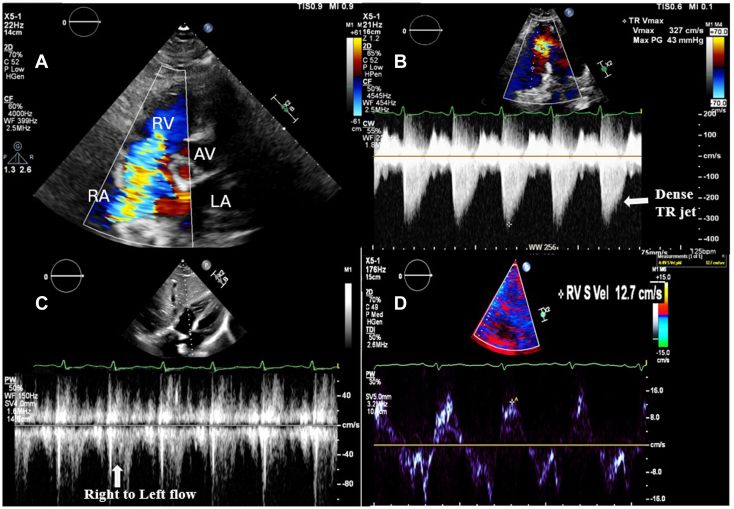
(A) Two-dimensional TTE, basal parasternal short-axis systolic view with color-flow Doppler, demonstrates severe TR; (B) continuous-wave spectral Doppler display demonstrates a triangle-shaped, dense profile (*arrow*) consistent with acute severe TR; (C) pulsed-wave spectral Doppler display of the interatrial septum demonstrates right-to-left Doppler flow profile (*arrow*); and (D) tissue Doppler spectral display of the tricuspid annulus demonstrates a myocardial systolic velocity profile, suggesting normal right ventricular function (12.7 cm/sec). *AV*, Aortic valve; *LA*, left atrium; *RA*, right atrium.
